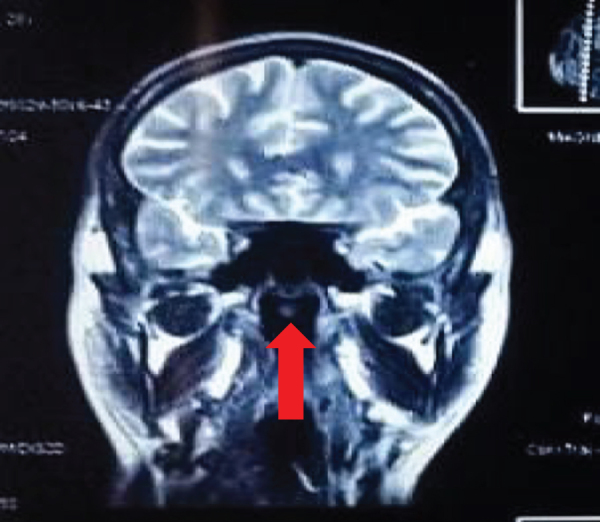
T2-weighted fat-suppressed fluid-attenuated inversion recovery image – coronal view of magnetic resonance scans, showing hyperintense rounded cystic area representing Thornwaldt’s cyst.
